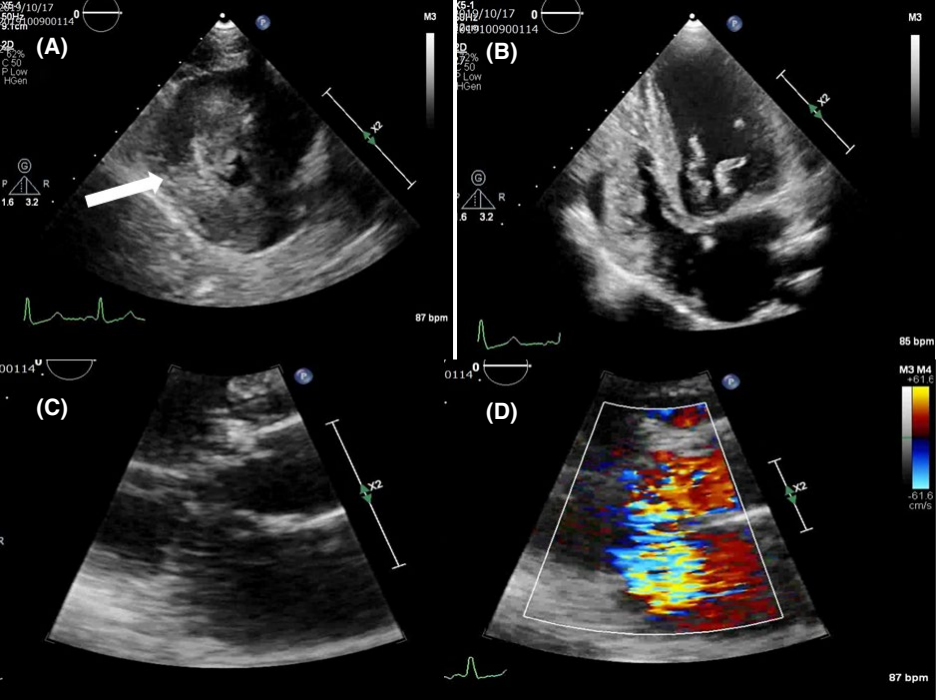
Preoperative transthoracic echocardiography images. A, The parasternal short‐axis view shows severe left ventricular hypertrophy (arrow). B, The apical 4‐chamber view shows degeneration and elongation of the mitral valve bileaflets. C, The B‐mode demonstrates systolic anterior motion of the mitral valve. D, A color Doppler demonstrates severe mitral regurgitation due to systolic anterior motion
